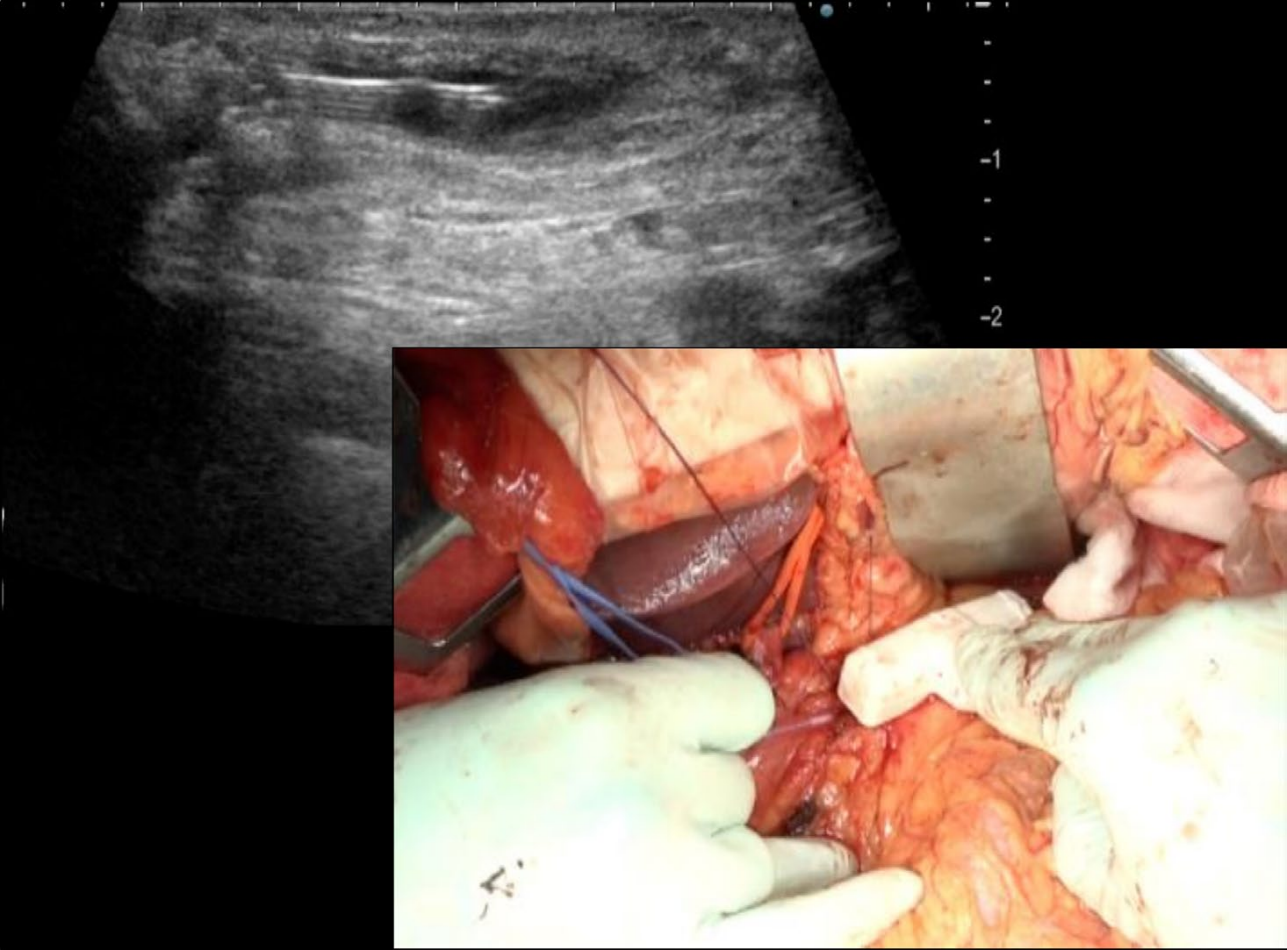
Intraoperative ultrasound‐guided puncture of the main pancreatic duct. The main pancreatic duct is being identified and punctured under real‐time ultrasound guidance to facilitate safe and accurate localization prior to longitudinal incision.
